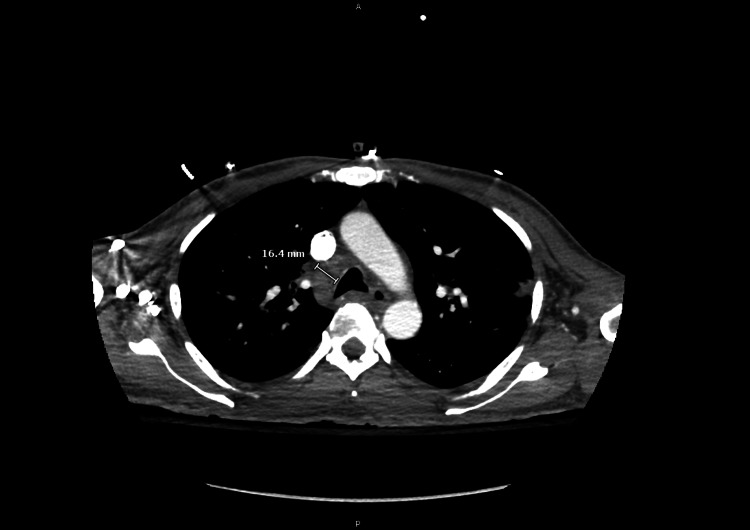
CTA of the chest demonstrating mediastinal and hilar lymphadenopathy. Image 1: CTA of the chest demonstrating mediastinal and hilar lymphadenopathy. The patient had been under outpatient pulmonology follow-up for waxing and waning pulmonary nodules. A bronchoscopy with endobronchial ultrasound (EBUS) performed one year prior revealed lymphohistiocytic elements, without evidence of malignancy. These findings were considered suggestive of an inflammatory process, with differential considerations including organizing pneumonia, hypersensitivity pneumonitis, chronic eosinophilic pneumonia vs. other autoimmune etiologies.
